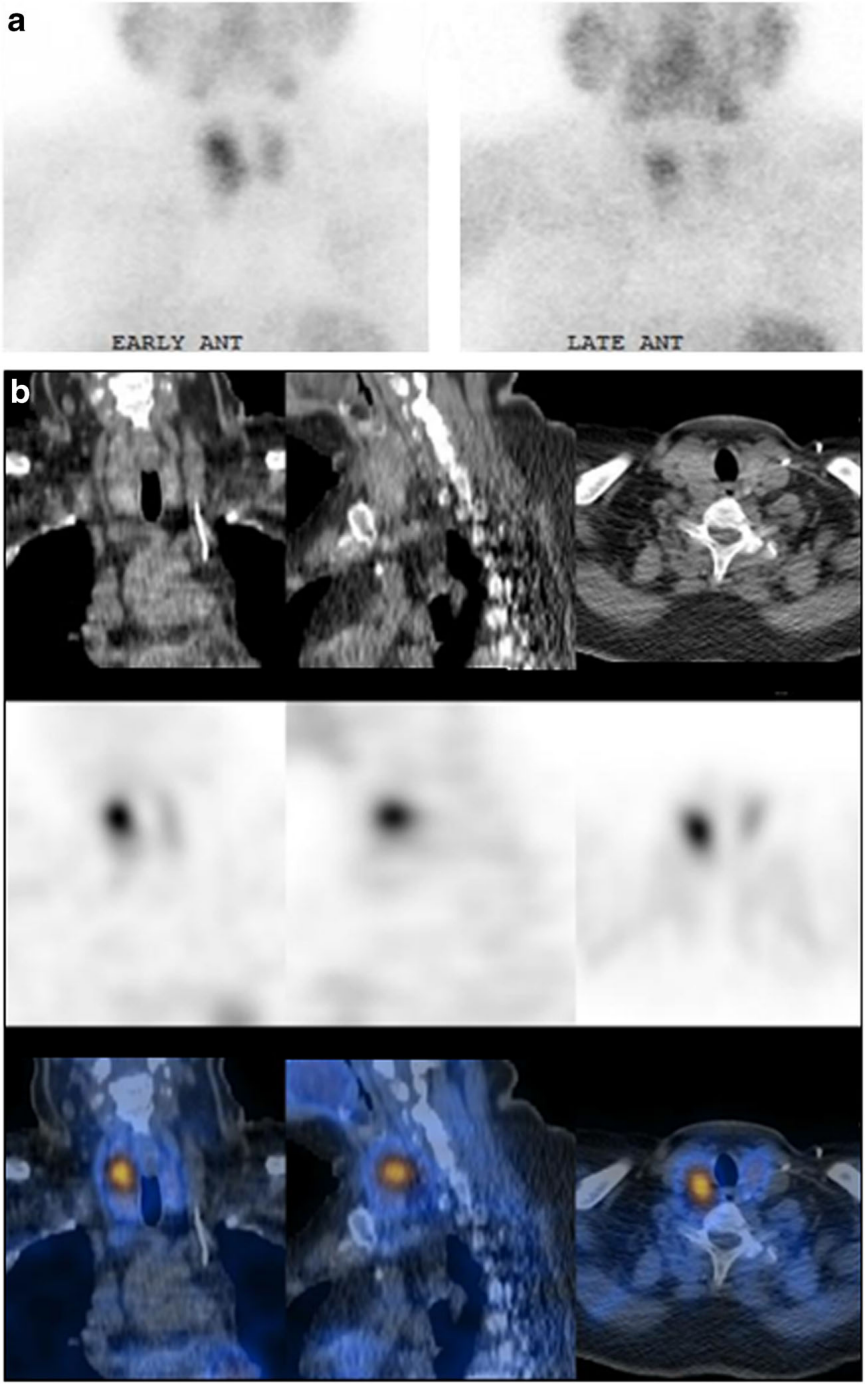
^99m^Tc-Sestamibi SPECT/CT for localization of parathyroid adenoma (PTA). A 57-year-old woman with laboratory evidence of primary hyperparathyroidism was referred for localization of PTA. Planar scintigraphy (a) shows an early area of increased focal uptake at the upper pole of the right thyroid lobe (left), with washout of the tracer from adjacent thyroid tissue on late images (right). SPECT/CT (b) performed 1 h after tracer injection localizes this focal uptake to a 16-mm nodule behind the thyroid gland, consistent with a PTA
